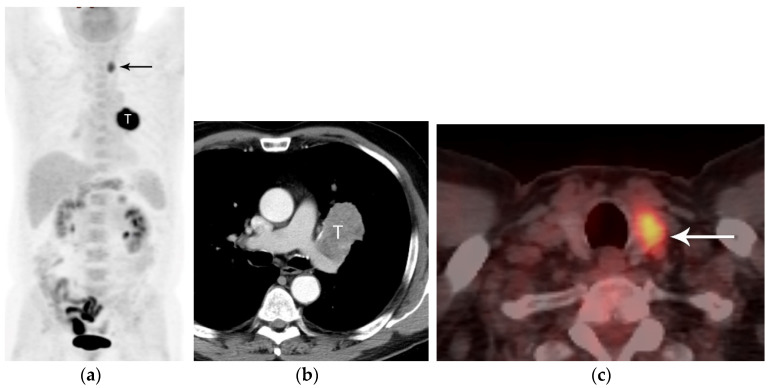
FDG-avid lesion unrelated to lung cancer. (a) Whole-body PET shows the left upper lobe primary tumor (T) and an FDG-avid focus in the left neck (arrow). (b) CT shows the left upper lobe tumor (T). (c) Axial PET/CT shows the FDG-avid focus is localized to the left lobe of the thyroid. Biopsy showed colloid nodule.
